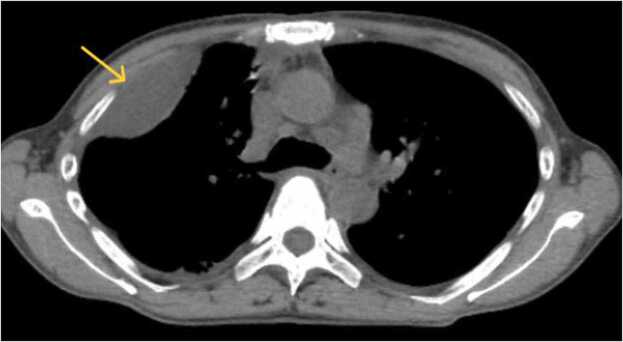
Follow-up CT on day fifteen after hospitalization. That revealed recurrent right pleural effusion in the right anterior space (yellow arrow).
